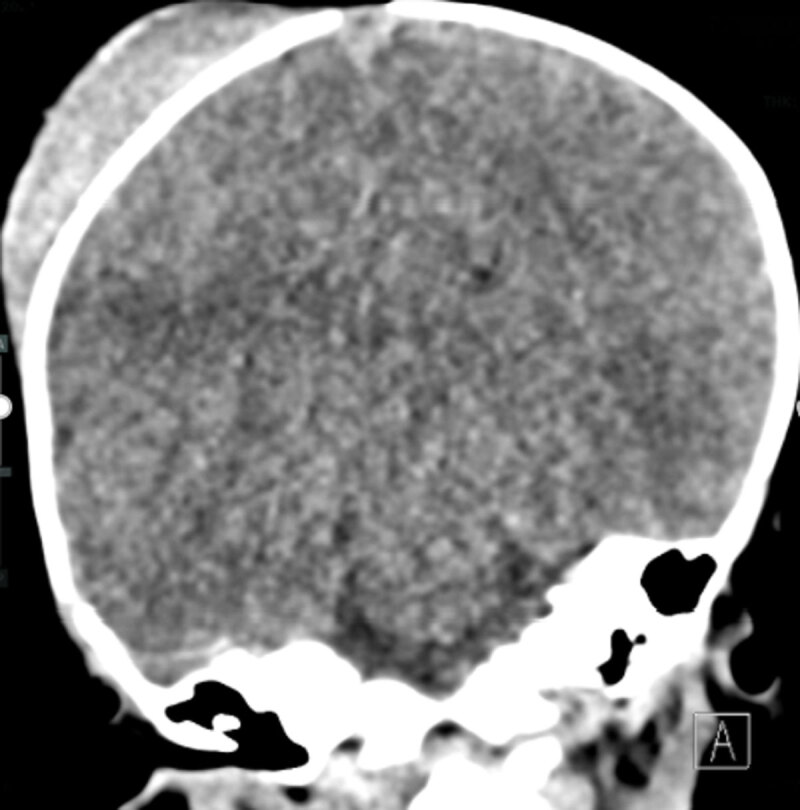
The ultrasound findings were attributed to the occurrence of a mirror-image artifact.
